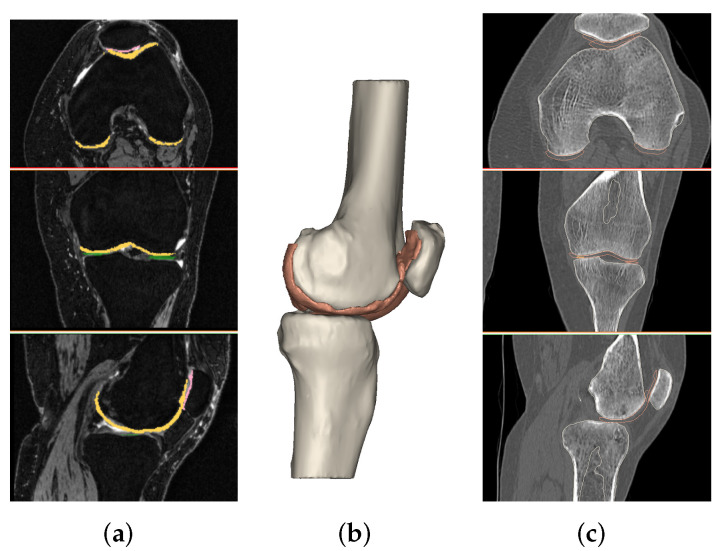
(a) Segmentation of femur cartilage from MRI file. (b) A 3D model of bones and cartilages. (c) Registration on CT file.
